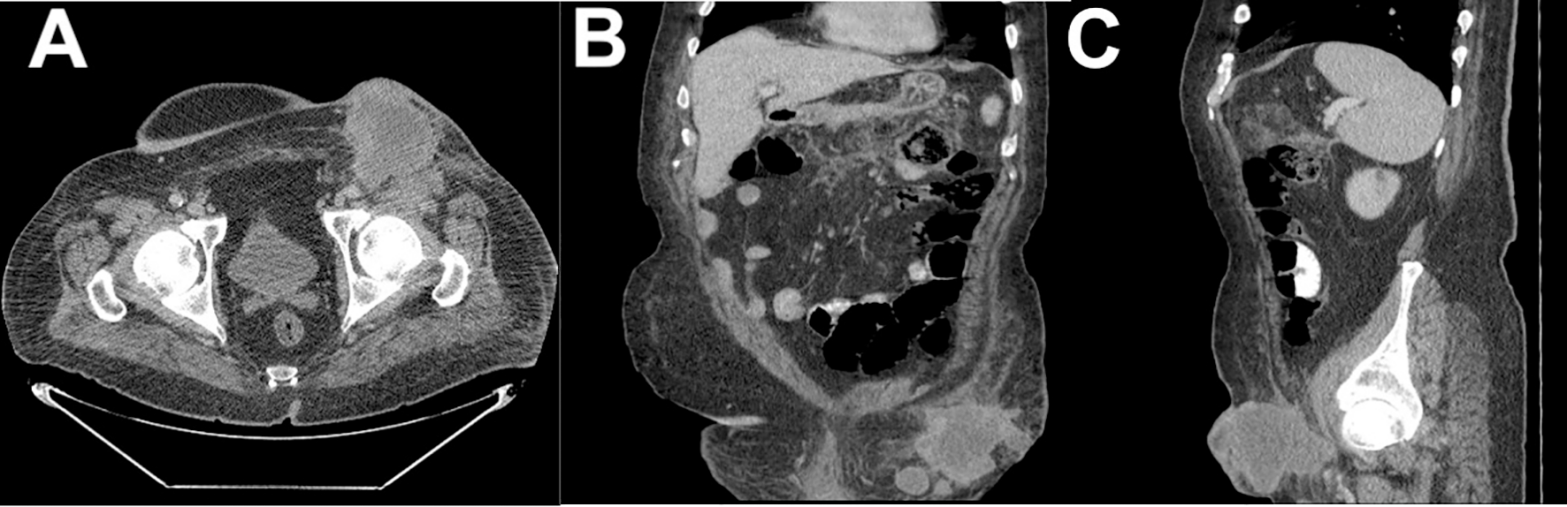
CT scan of the left inguinal mass: (A) axial plane view; (B) coronal plane view; and (C) sagittal plane view.
